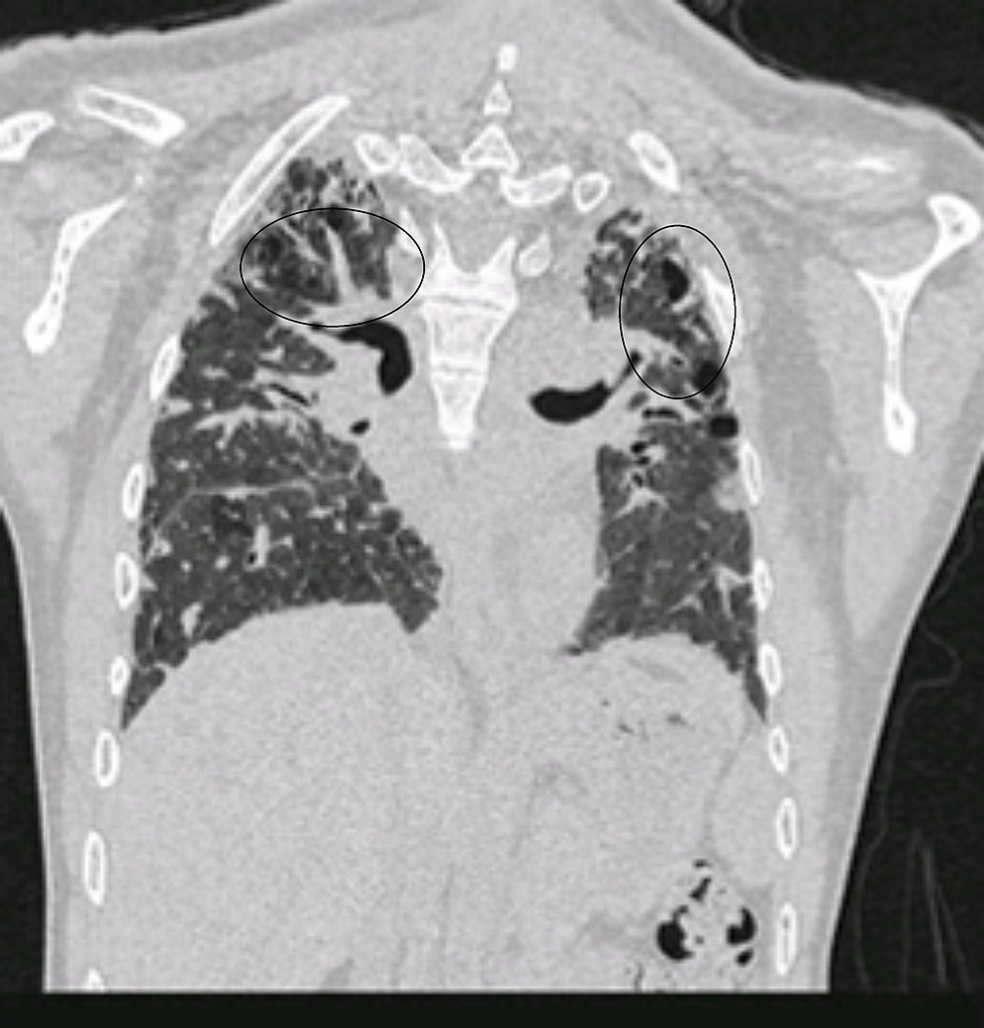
Coronal cut of high-resolution CT in 2018. Segmental areas of consolidation collapse were seen in both lung fields, especially in the posterior segment of the right upper lobe.
